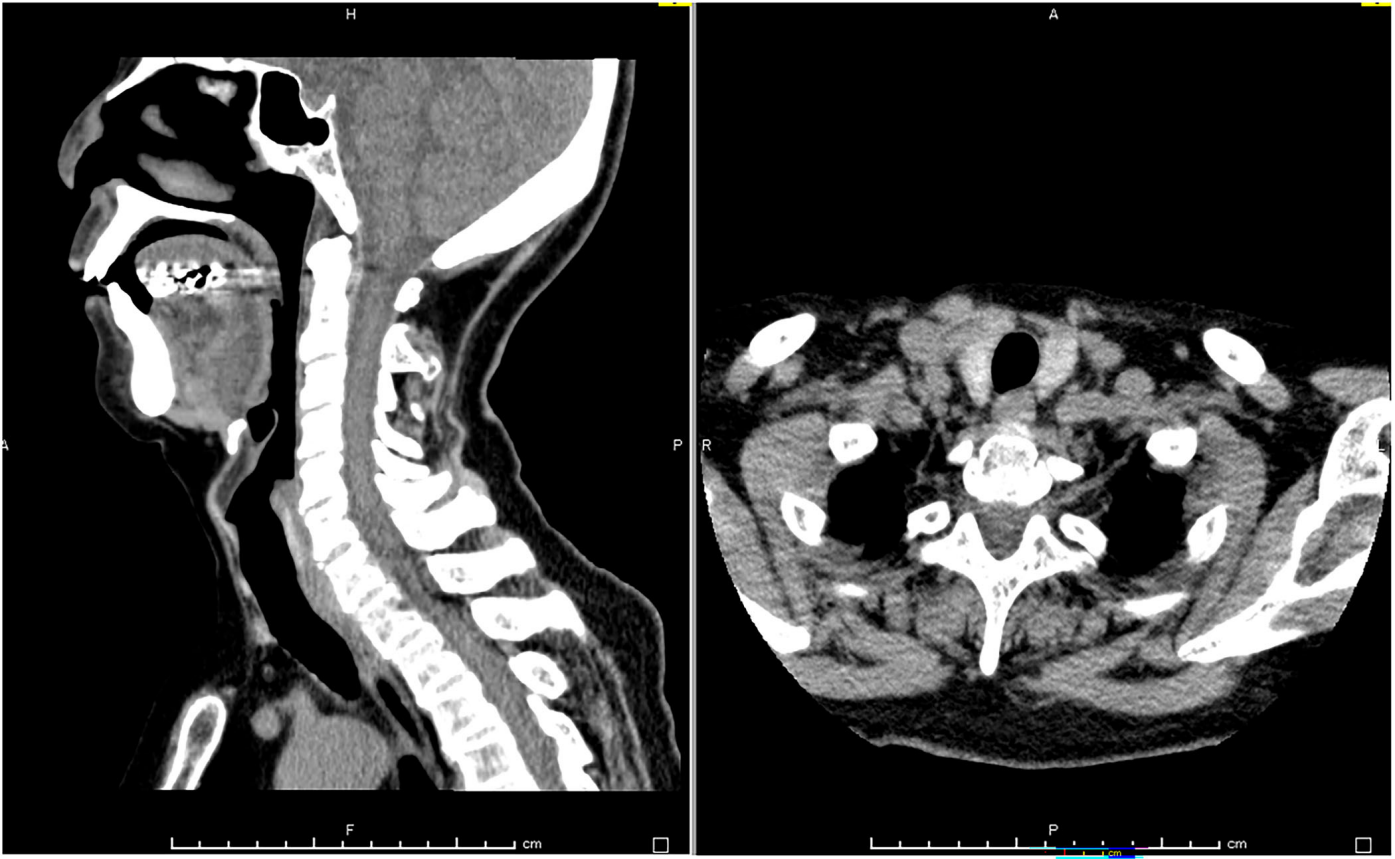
The computed tomography scan showed no tumor or other organic disease causing the snoring.
